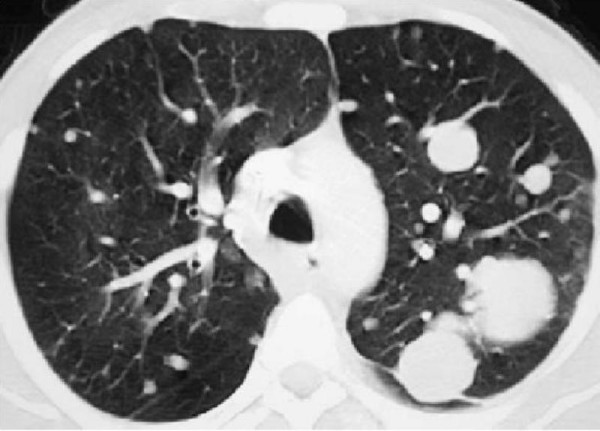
CT scan view of pulmonary metastasis.
